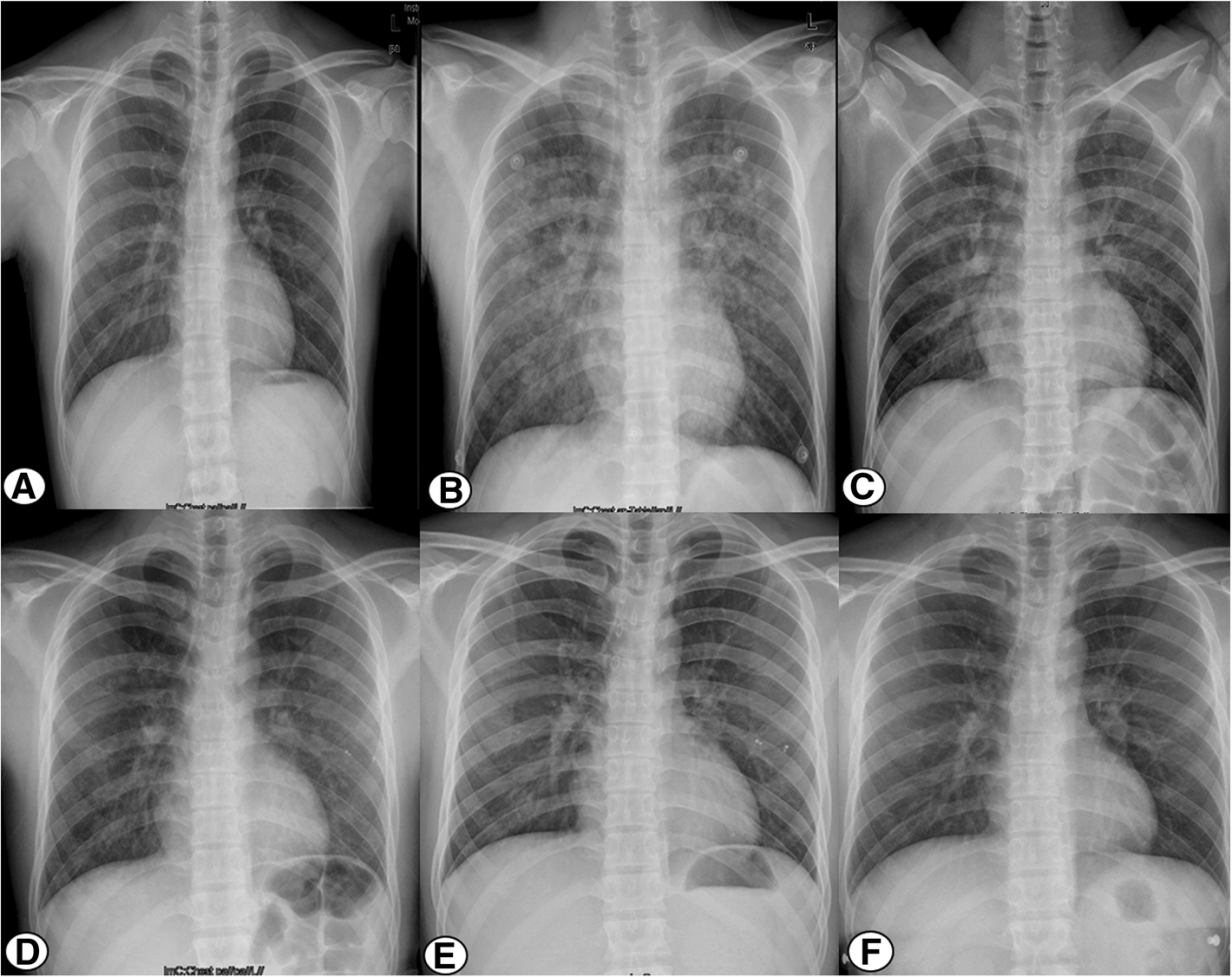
a-f chest radiograph obtain preoperative, 2 h, 17 h, 23 h, 3 days and 6 weeks after the surgery. It was shown that bilateral infiltrates with opacities and nodules gradually decreased over time and completely disappeared on follow up
